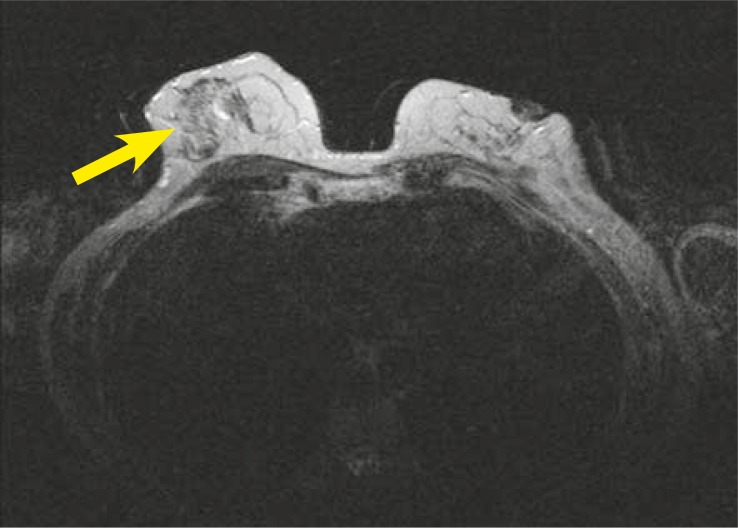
Axial T2-weighted image, a hiperintensive signal in the right breast (arrowhead).
